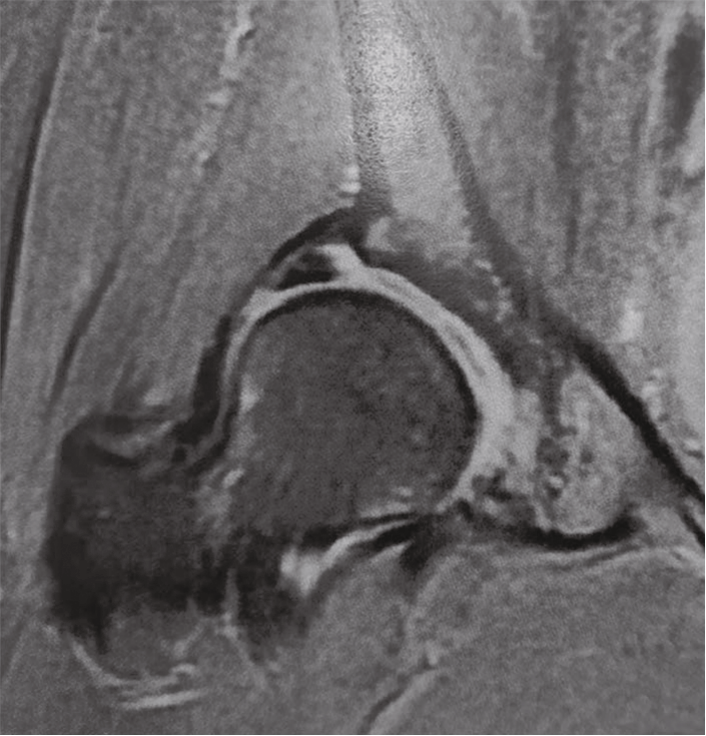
MRI (coronal cut) of the right hip showing dysplasia and a hypertrophic labrum torn at its base.
